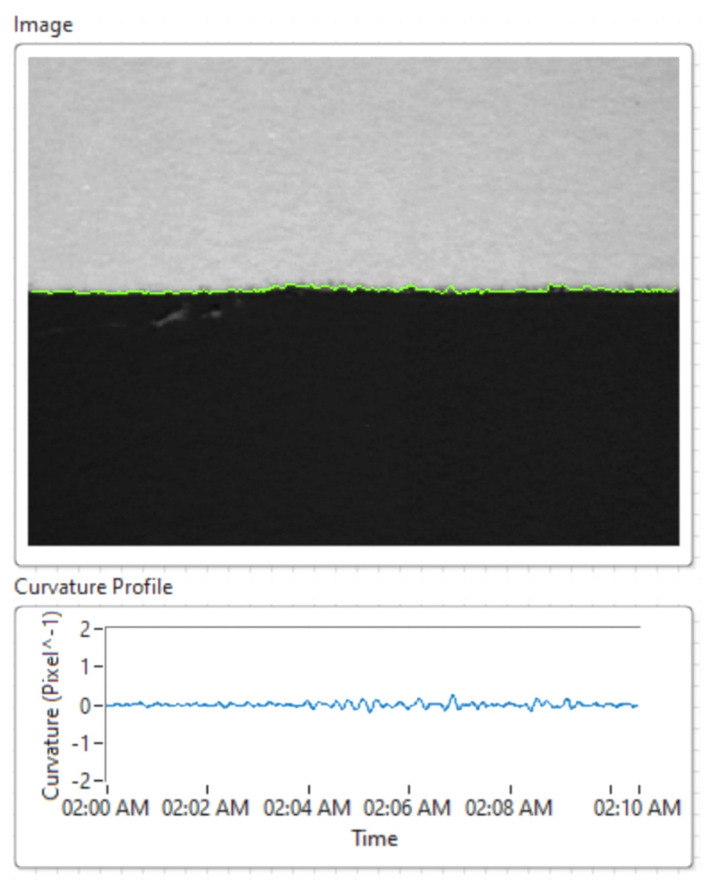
Determining the mechanical deformations of rails based on ultrasonic sensors.
